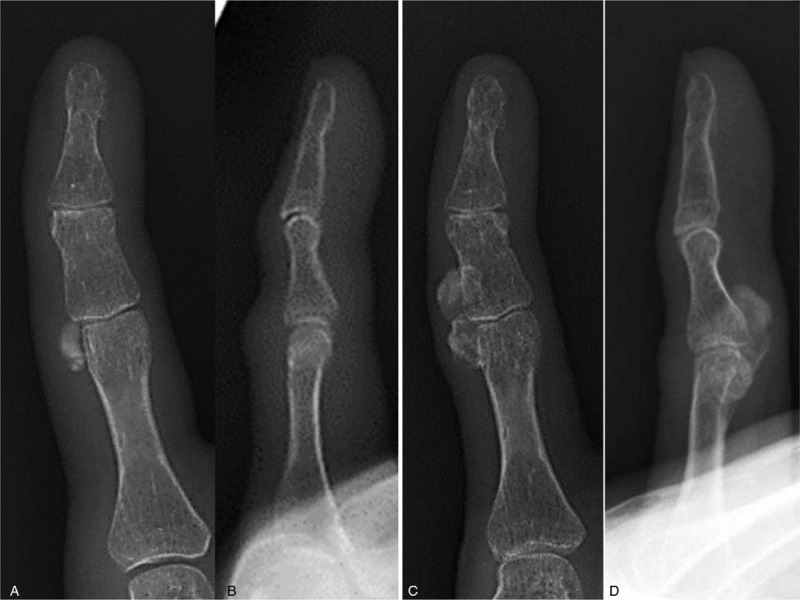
Plain (A) anteroposterior and (B) lateral radiographs taken at a neighboring clinic, showing a subcutaneous calcifying lesion (0.6 cm × 0.2 cm) on the ulnar side of the proximal phalangeal head of the left fifth finger. Initial presenting (C) anteroposterior and (D) lateral radiographs taken at our hospital, showing a 2-humped subcutaneous calcifying lesion (1.3 cm × 0.5 cm) on the ulnar side of the proximal interphalangeal joint of the left fifth finger.
